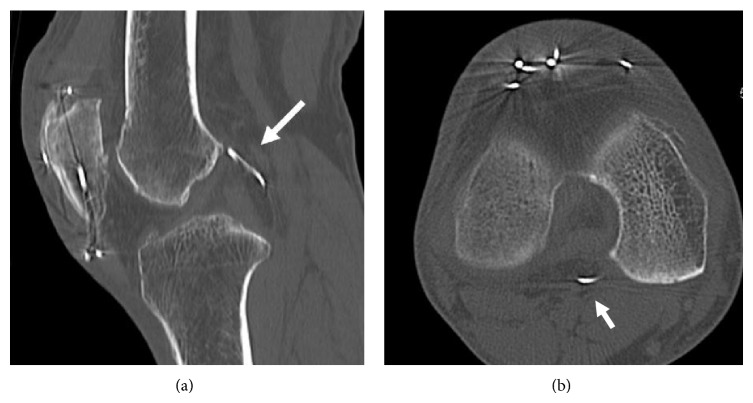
Computed tomography, (a) sagittal and (b) axial views. We could not determine from the scan whether the wire fragment was in the posterior compartment of the knee joint or popliteal fossa.
